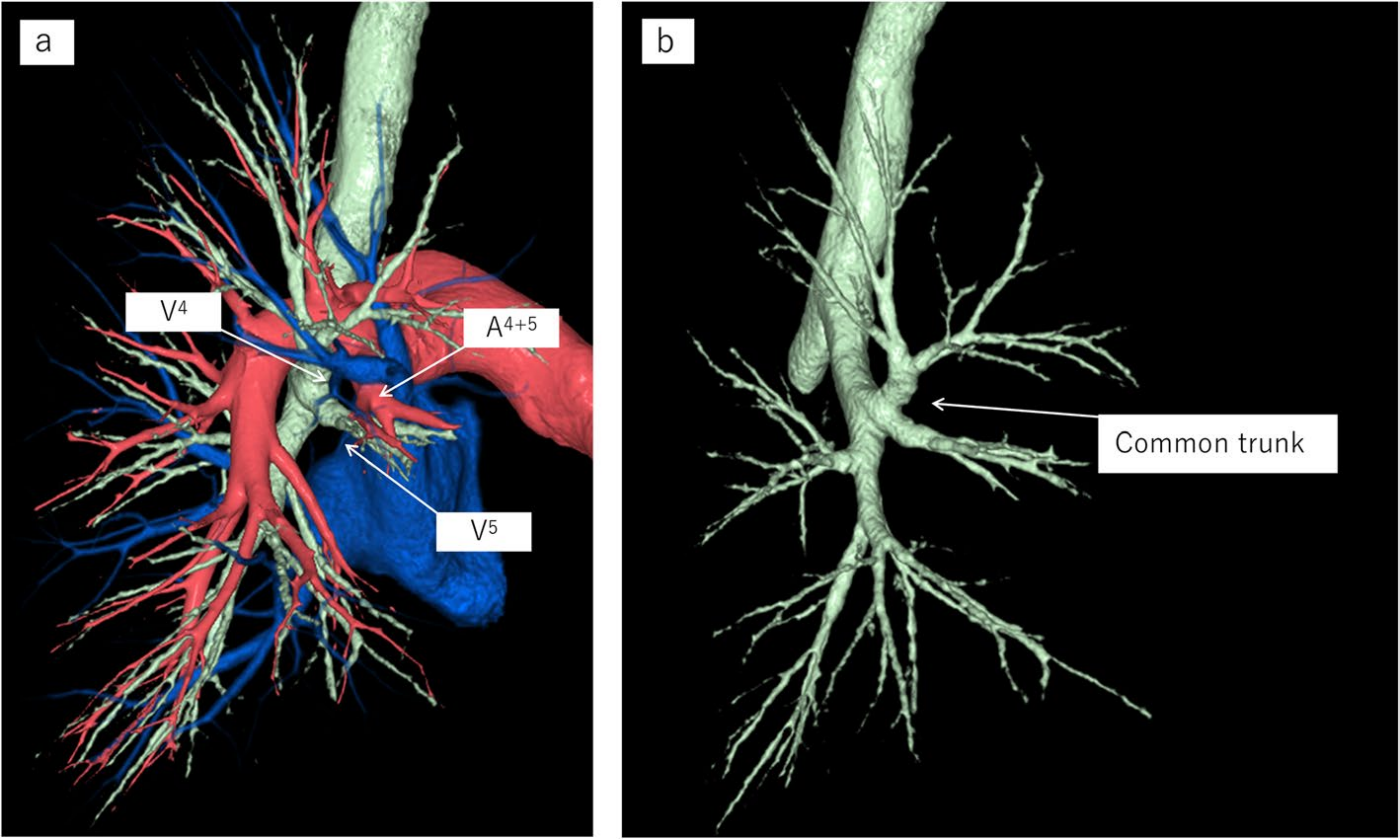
3D-CT revealed that the upper and middle lobar bronchi formed a common trunk with the mediastinal type of the right pulmonary artery. The arrows show the pulmonary artery and vein of the middle lobe. An anomalous A.^4+5^ was branched from the main pulmonary artery behind the superior pulmonary vein (a)
